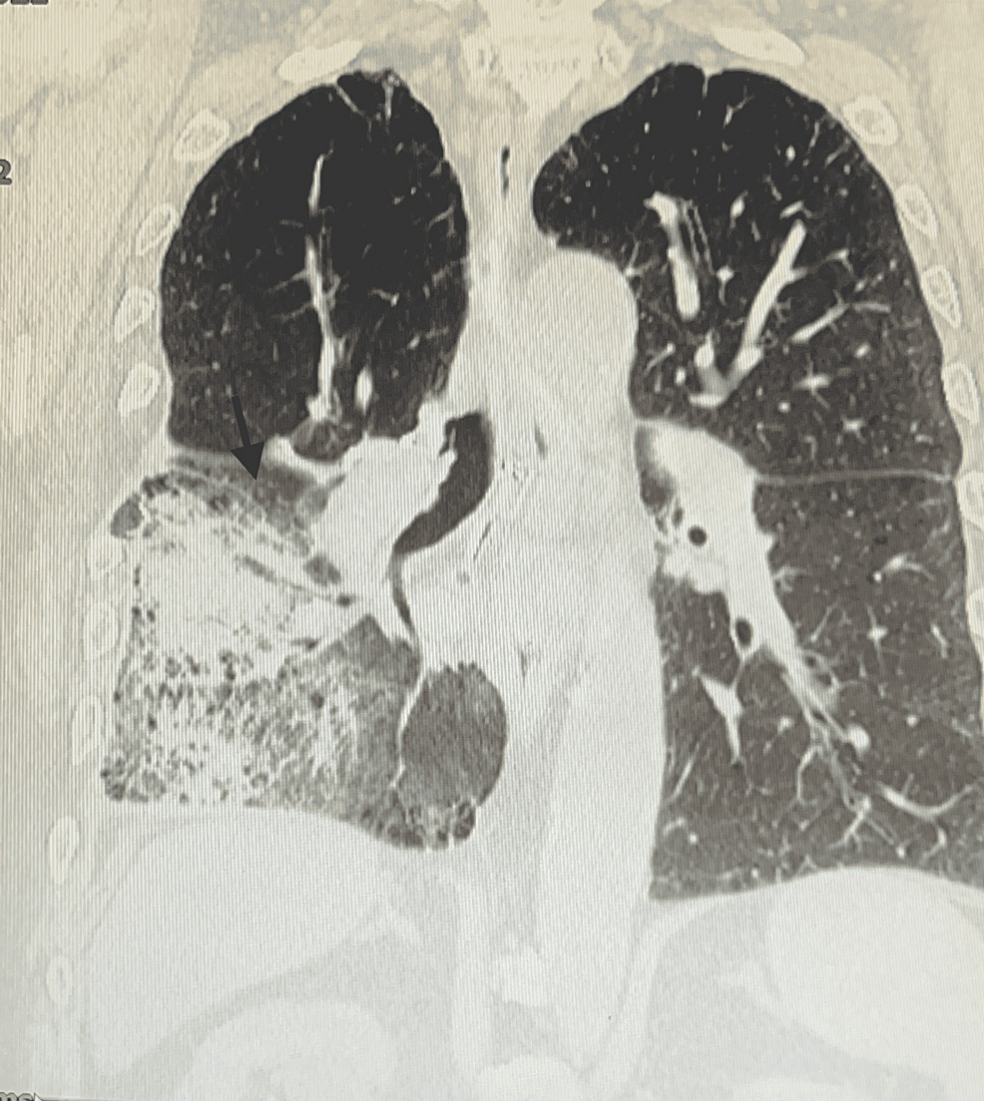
Chest CT demonstrating right lung opacity and infiltrating mass Arrow indicates the infiltrate
